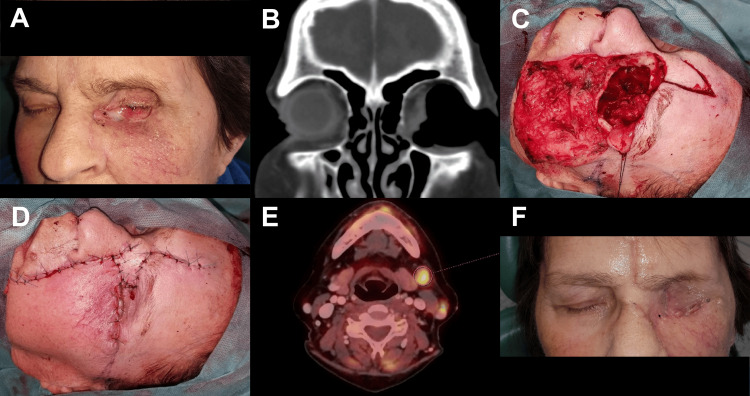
Poorly differentiated sebaceous carcinoma with perineural tumour propagation (A) Clinical state post three non-radical left orbital tumor removals including eyeball enucleation (R1), three months after surgical intervention (B) CT coronal section showing tumor osteolysis of the medial wall of the left orbit (C) post en bloc orbitectomy of the left orbit (medial and inferior wall), R1 resection with completion of resection during the operation (D) reconstruction only with local skin flaps due to the need for a shortened surgical procedure due to polymorbidity (i.a. Coronary artery disease of a transplanted heart) (E) F-18 fluorodeoxyglucose (18F-FDG) positron emission tomography (PET)/CT axial sections showing suspicion of two lymph node metastases in the left neck, not presented at the time of diagnosis, no further treatment options due to ongoing complicated suppurative meningitis, died of other causes (DOC) five months after surgery (F) post-operative healing, followed by adjuvant radiotherapy of the left orbit.
